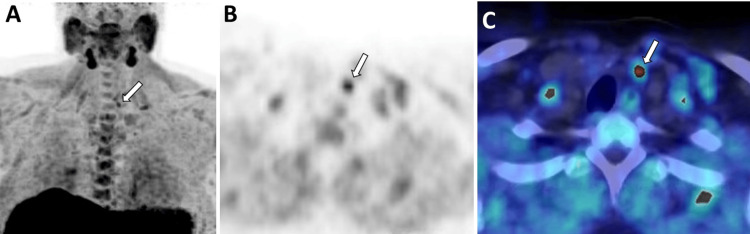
18F-Fluorocholine PET/CT. (A) Maximum intensity projection (MIP), (B) unfused axial PET, and (C) fused axial PET/CT images after an injection of 4.01 mCi of 18F-Fluorocholine display a subcentimeter focus of increased tracer uptake (SUVmax 4.5) in the left paraesophageal superior mediastinum (white arrow), consistent with a parathyroid adenoma.
